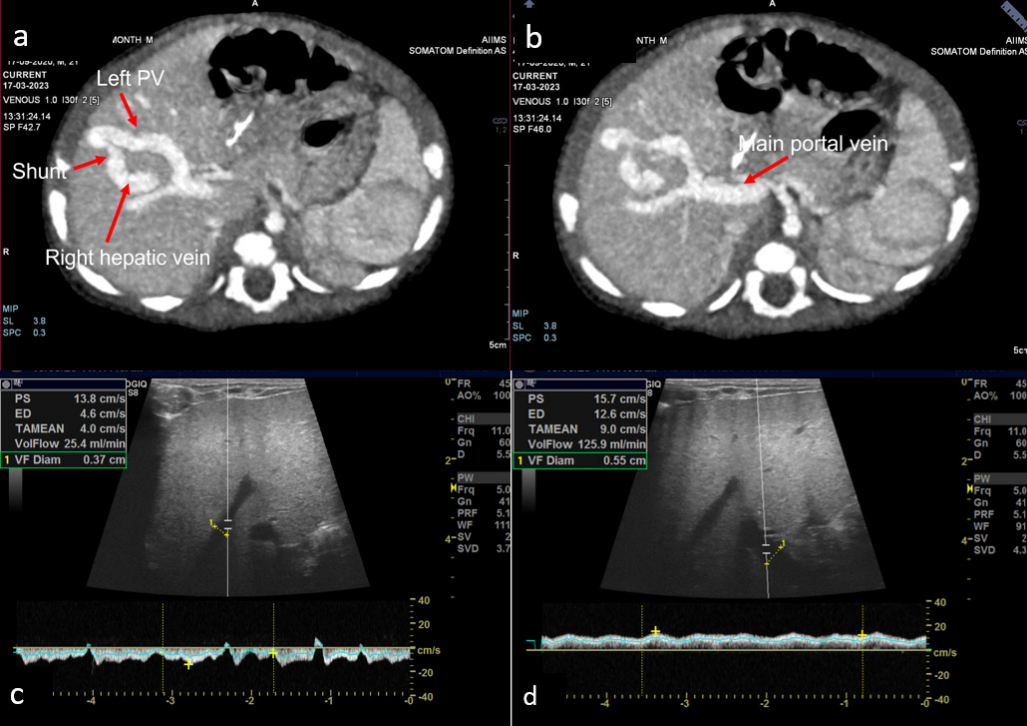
a) USG shows anechoic tubular structure communicating between left portal vein and right hepatic vein. b) On color Doppler there is communication between hepatic vein and portal vein which shows color flow with aliasing. c) Calculation of porto-venous shunt ratio is shown – ~88% in present case.
